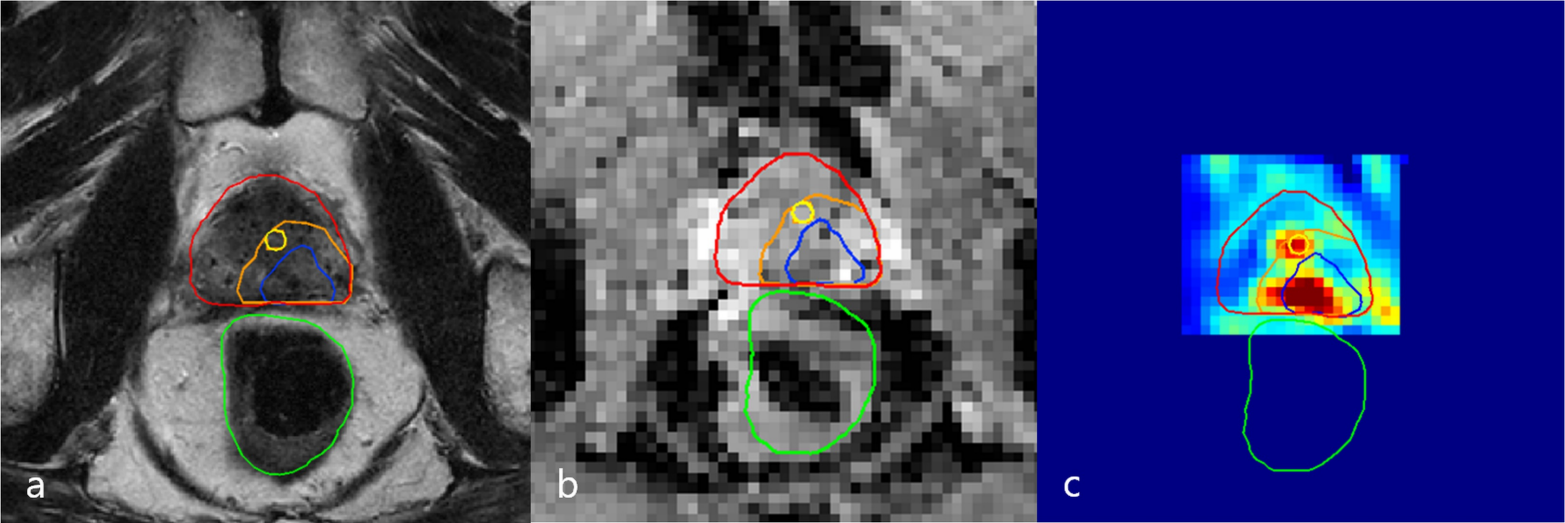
Diagnostic 3T mp-MRI revealing second recurrence lesion Transversal plane of the diagnostic 3T mp-MRI revealing a suspect lesion in the left dorsal peripheral zone. Delineations of the urethra (yellow), rectum (green), prostate (red), gross tumor volume (GTV, blue), and clinical target volume (CTV, orange) are shown. (a) T2-weighted image, (b) ADC image, (c) K-trans image mp-MRI: multiparetic magnetic resonance imaging
